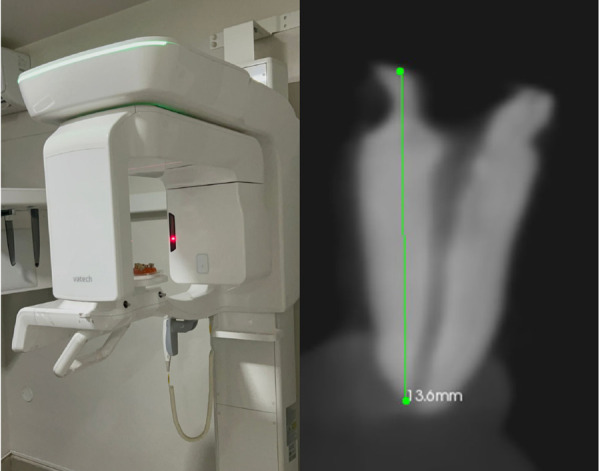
Determinación de la longitud en un corte sagital con el auxilio de un CBCT.
